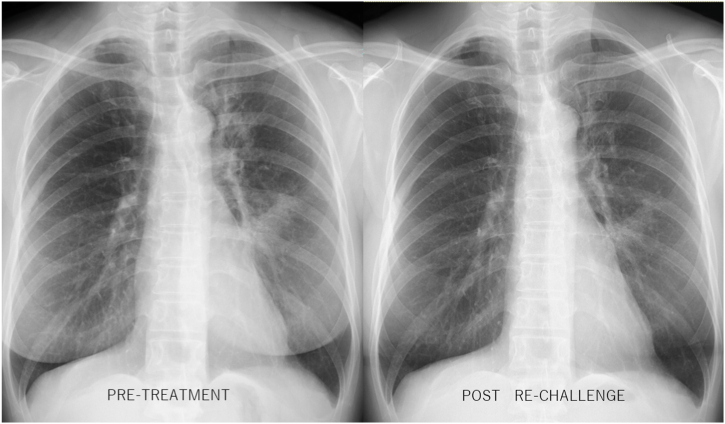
Radiographic response to zongertinib. Chest radiographs showing significant reduction of the lung tumor following the successful re-challenge of zongertinib.
